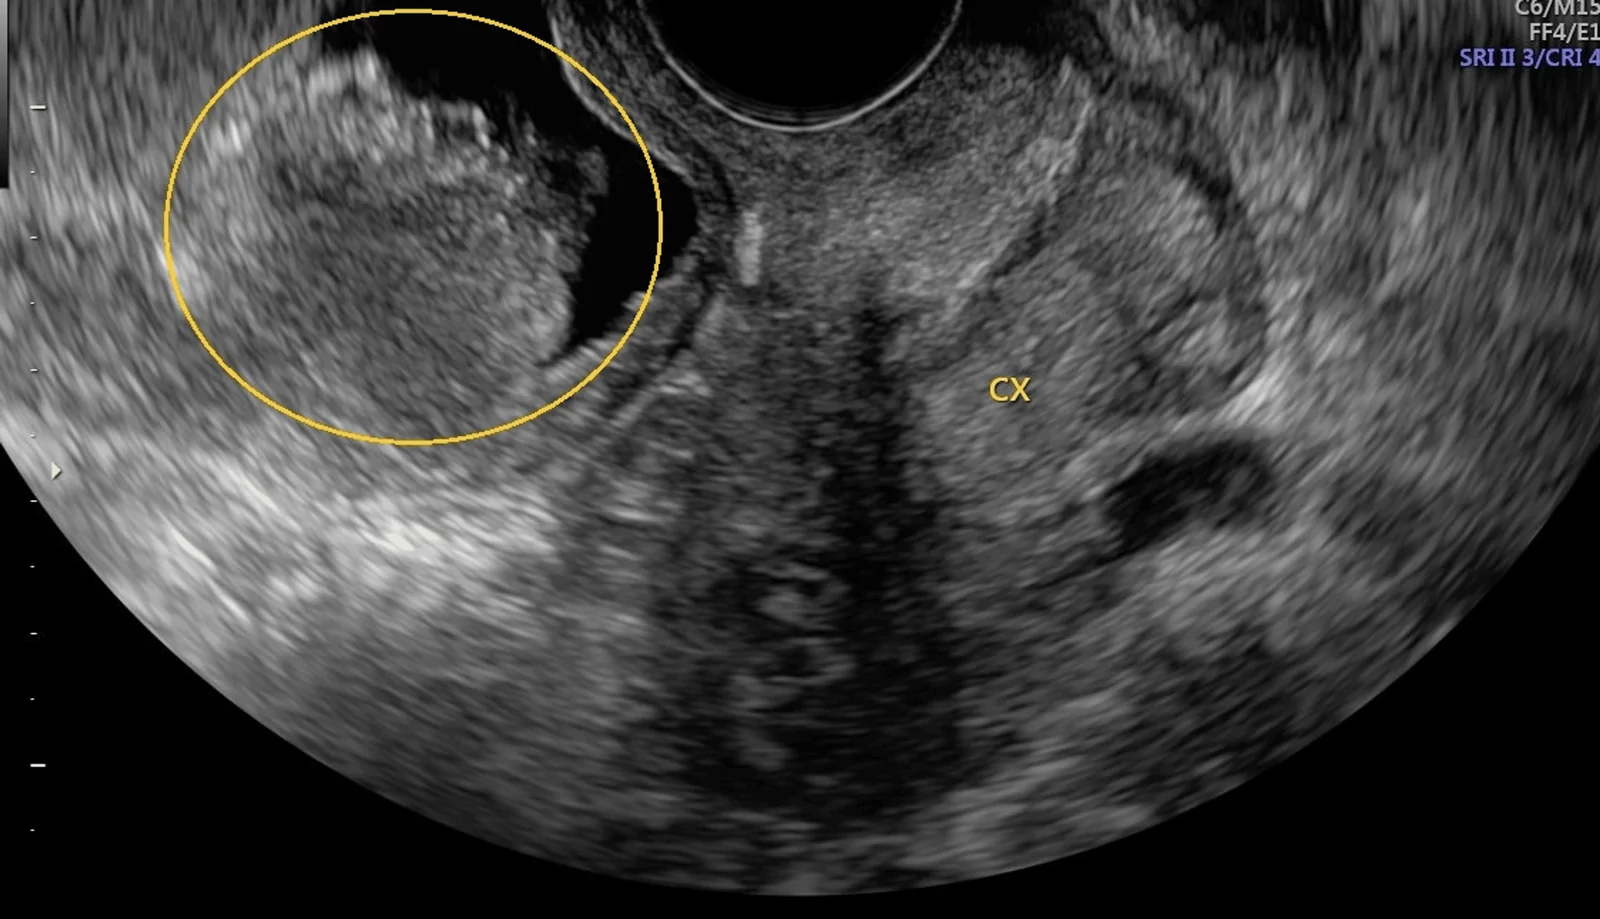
Ultrasound pelvis suggestive of a heterogeneous mass possibly of uterine or adnexal pathology as indicated by the circle.
